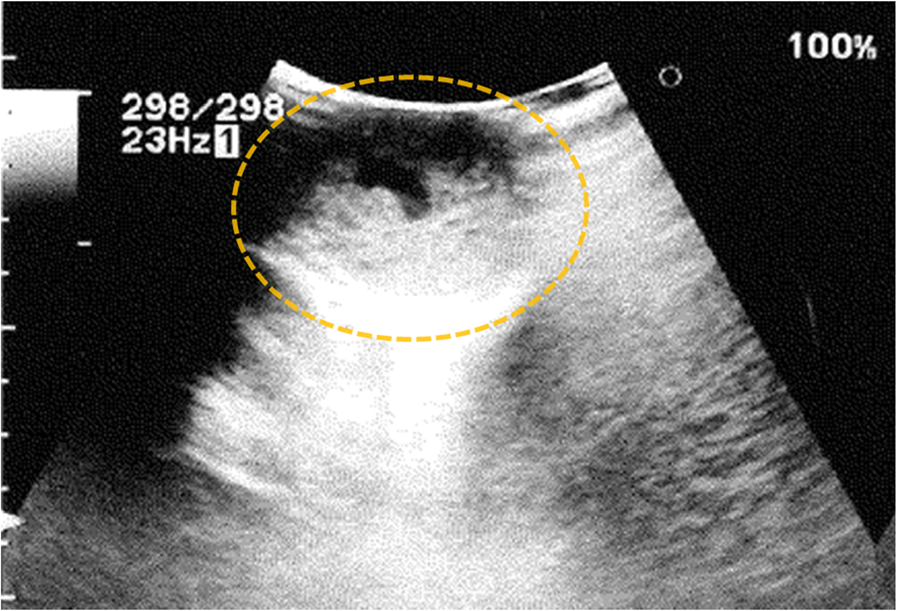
Ultrasonographic findings in the patient. Dotted circle indicates a mass with a heteroechoic pattern and 4-cm diameter
